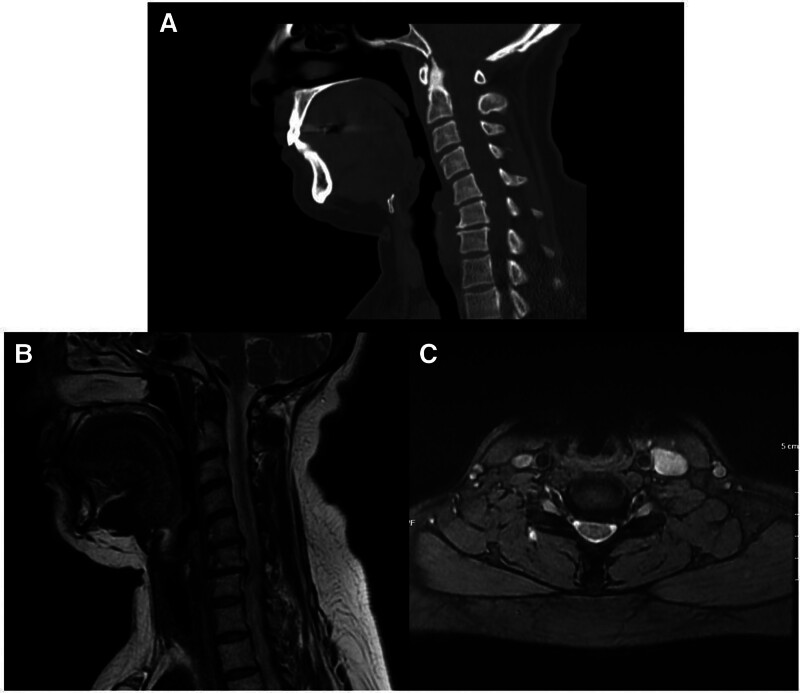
Cervical CT sagittal image showing cervical kyphotic alignment (A), sagittal, (B) and transverse (C) section of cervical MRI of the patient. Broad-based posterior disc protrusion at C5–C6 level, narrowing in anterior subarachnoid space. Left posterior protrusion at C6–C7 disc, compression of the cervical ganglion within the left neural foramen. CT = computed tomography, MRI = magnetic resonance imaging.
